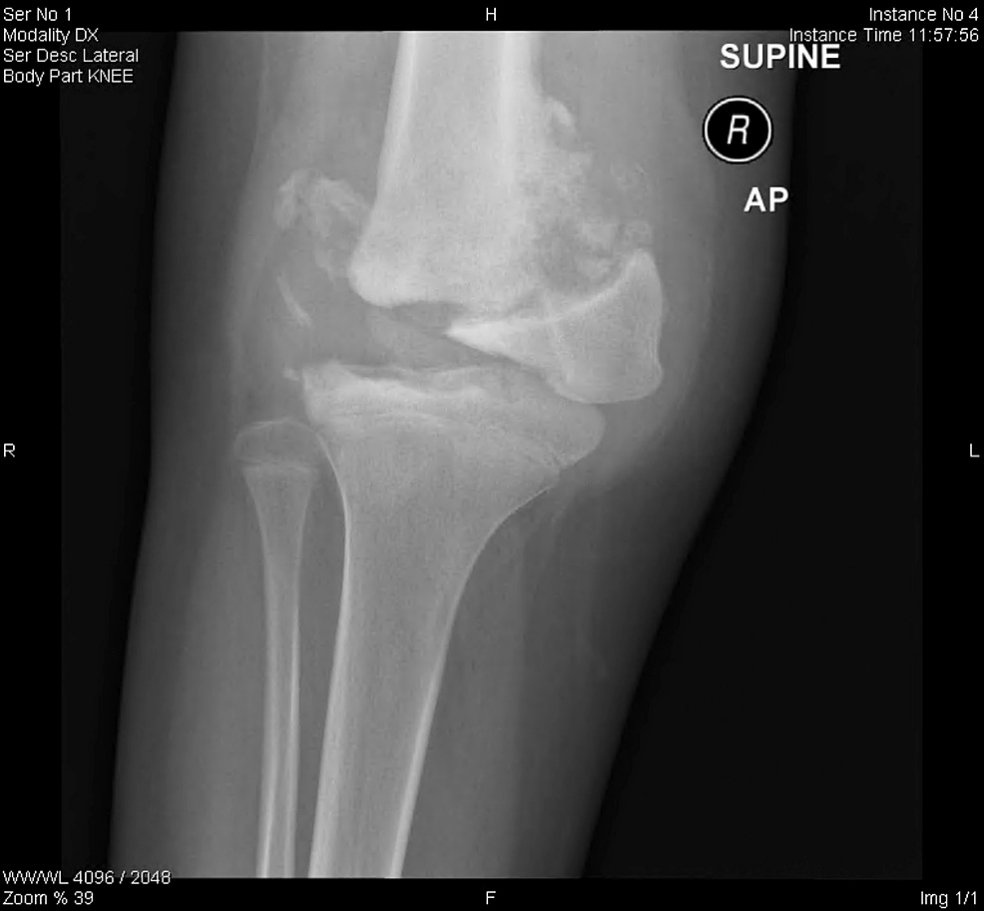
X-ray (anteroposterior view) of the right knee.
